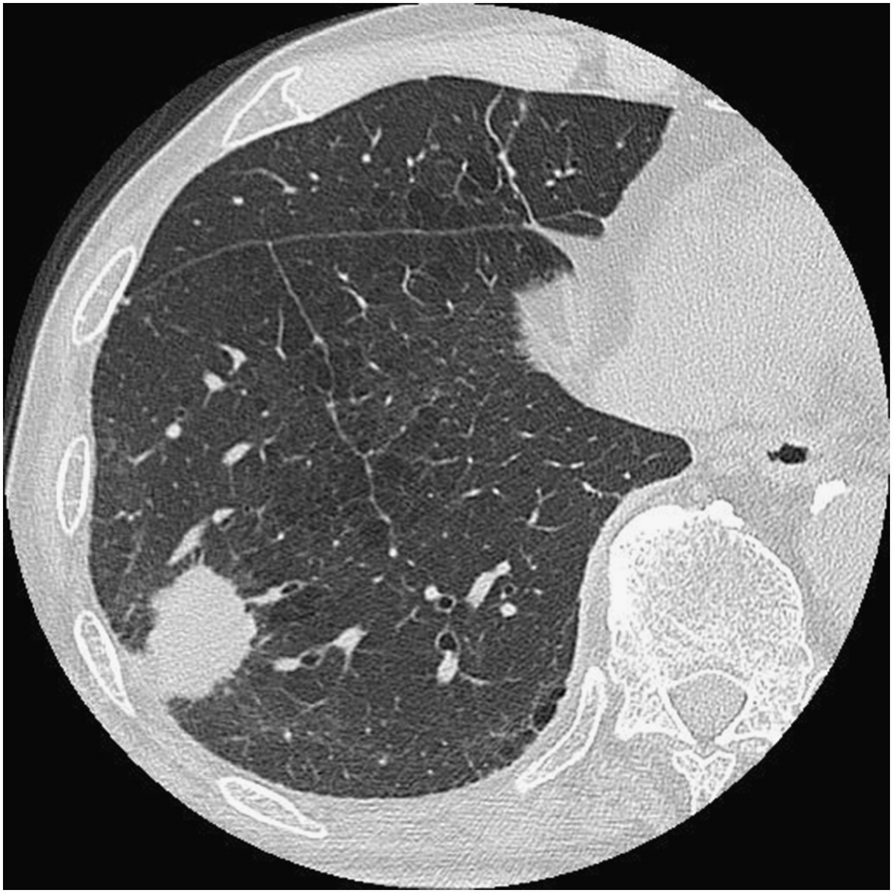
Chest computed tomography scan showing the shadow of a solid mass with spiculation in the patient’s right Segment 9b region, and emphysematous lung.
